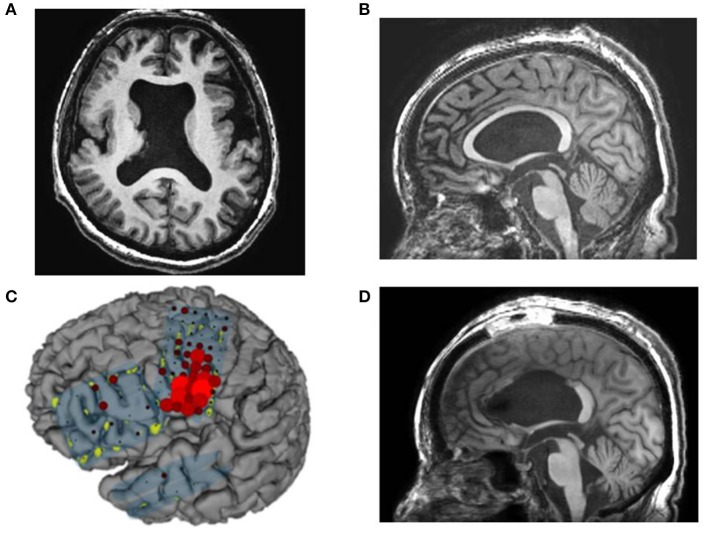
Case 14: (A,B) Preoperative magnetic resonance imaging showing cortical dysplasia and thin corpus callosum, (C) high gamma activity (HGA) mapping with an overt word reading task showing accumulation of HGA mainly on the mouth primary motor area (PMA). (D) Postoperative magnetic resonance imaging showing resection of the anterior and middle corpus callosum except for the splenium.
